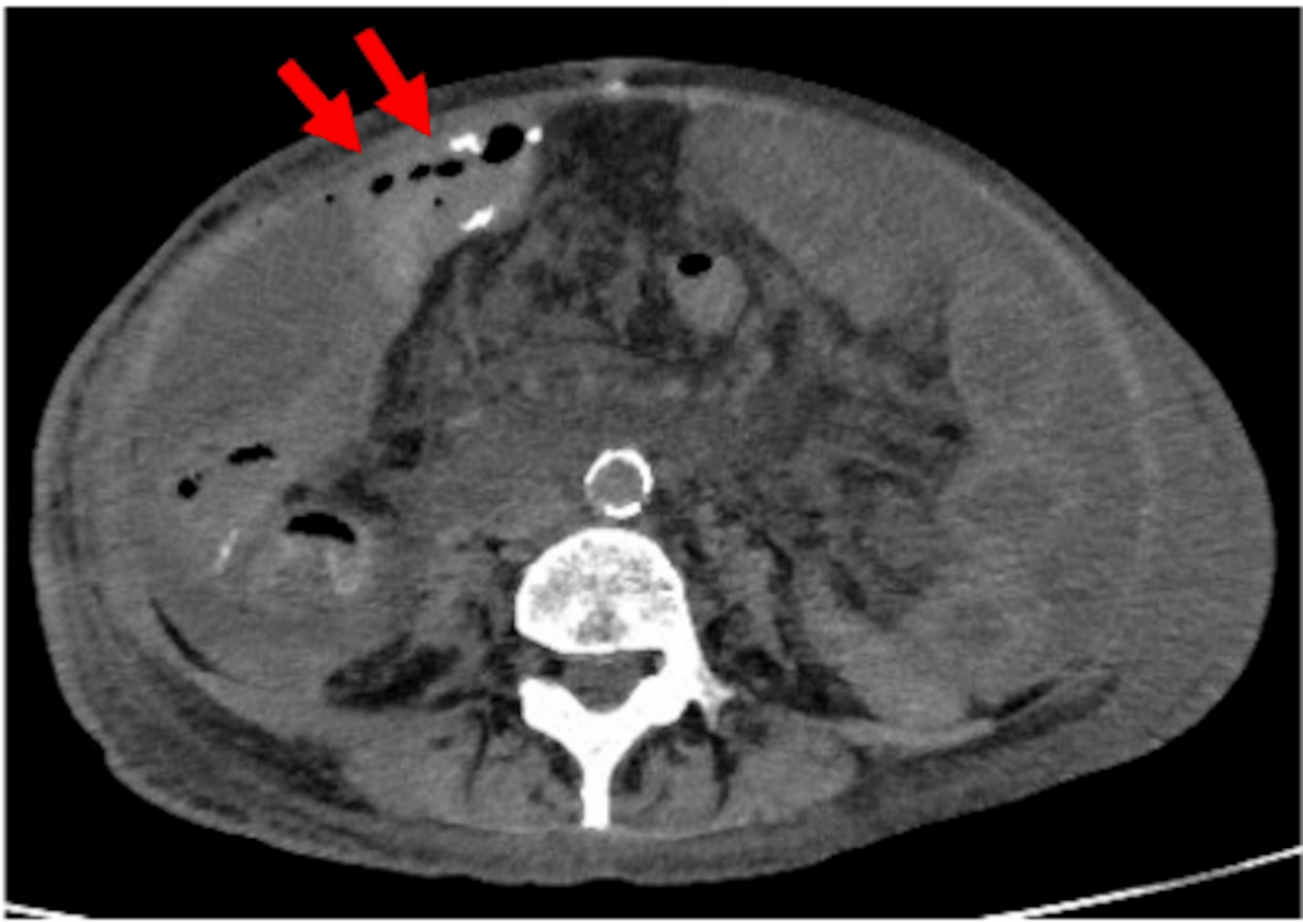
Computed tomography image on postoperative day 15 Free air was observed around the transverse colonic anastomosis, leading to the diagnosis of anastomotic leakage (→)
